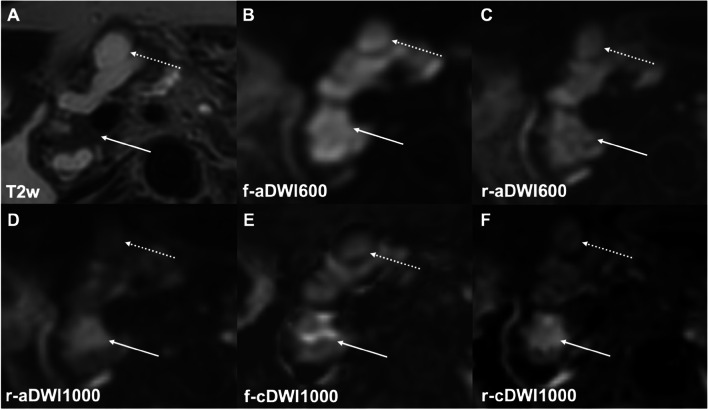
A mixed-type IPMN in a 75-year-old female patient. The solid tissue component in the pancreatic head (T2w, arrow) is not visible in both conventional and high-resolution DWI at *b* = 600 s/mm^2^ (B, C) and led to the false diagnosis of a benign IPMN. Also, the conventional high-*b*-value computed image indicates no clear diffusion restriction (D). However, the high-resolution high-*b*-value computed image (E) led to the correct diagnosis of malignant IPMN, which was confirmed pathologically after resection. Fluid suppression (dotted arrows) is best achieved in r-cDWI1000. Furthermore, high-resolution high-*b*-value computed DWI outperforms directly acquired high-resolution high-*b*-value DWI (F) regarding image quality and fluid suppression
